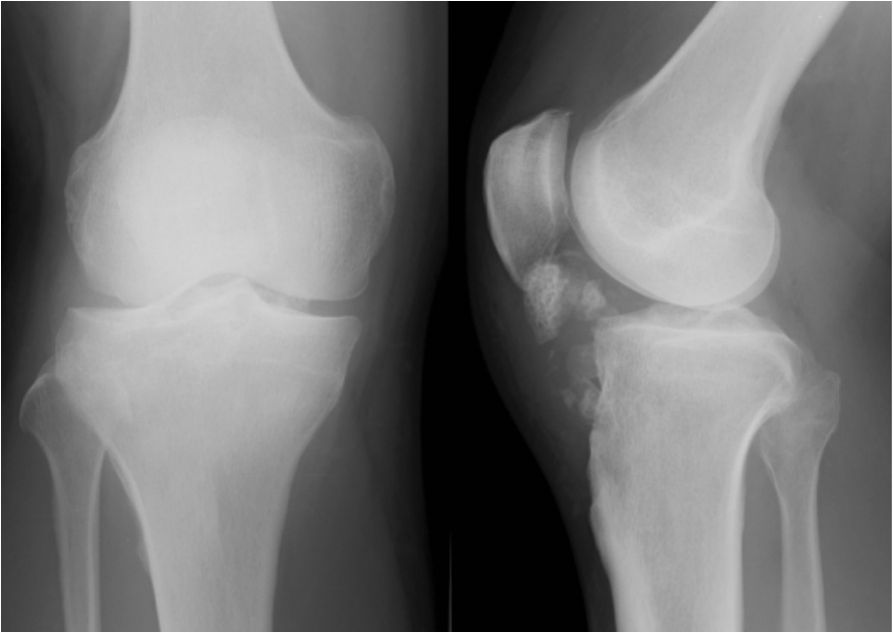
Preoperative radiographs of the left knee showed multiple ossifying tumors at a site consistent with the infrapatellar fat pad.
